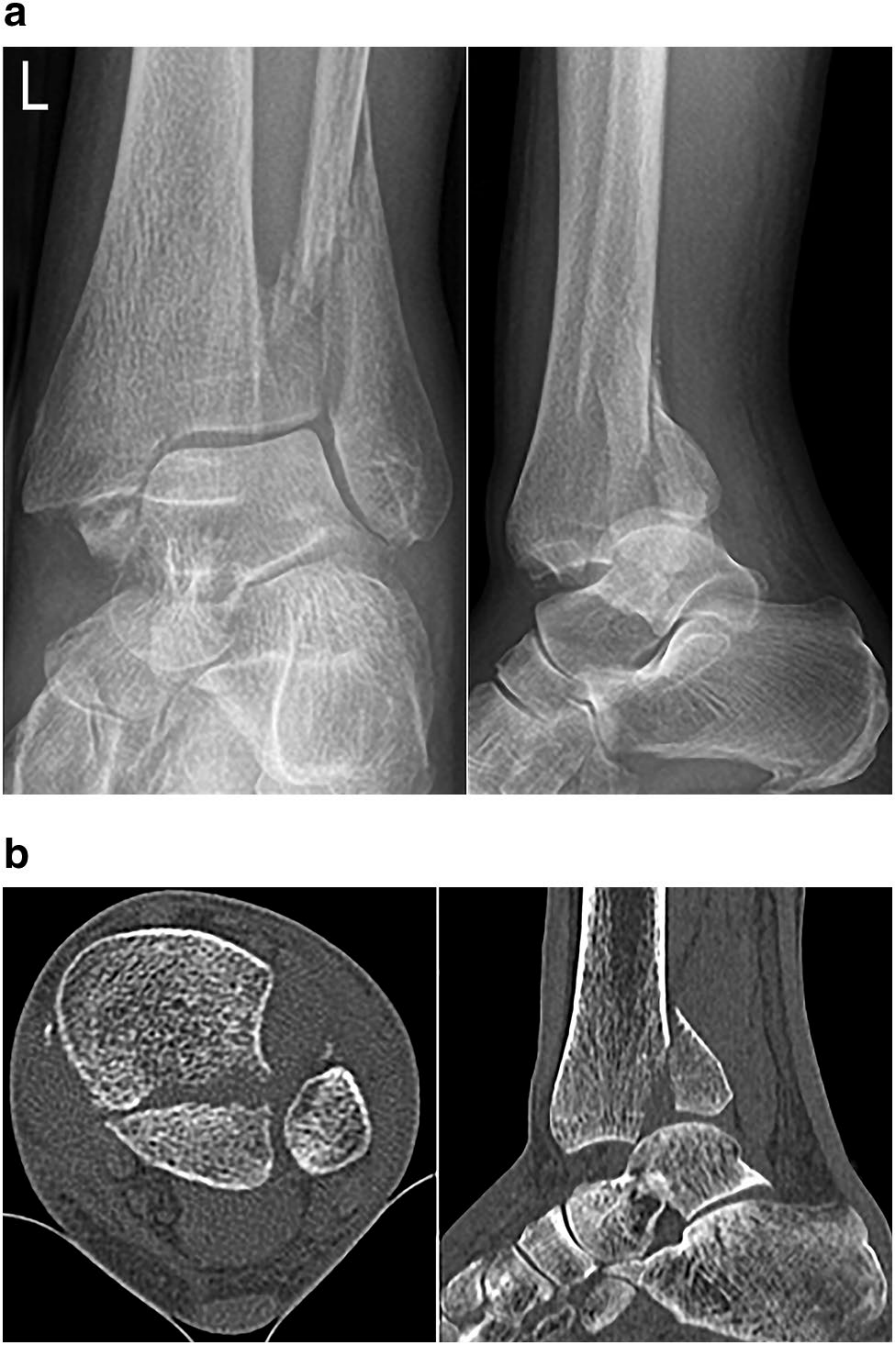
a Preoperative anteroposterior and lateral radiographs of a 74-year-old female patient with a quadrimalleolar fracture-dislocation of her left ankle. b Preoperative axial and sagittal CT images reveal a large triangular posterior malleolar fragment (Bartoníček–Rammelt type 4) and a chip-like avulsion from the anterior tibial tubercle
